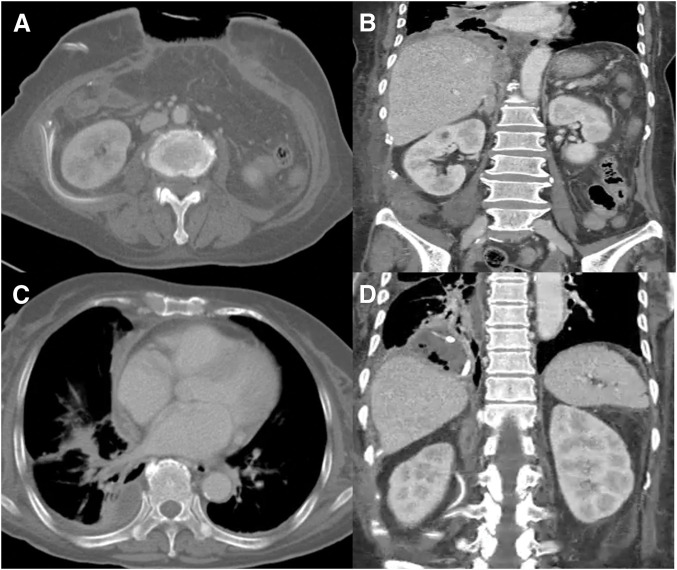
Follow-up CT images demonstrating interval improvement of the infectious process. Marked decrease in fluid collection at the operative site (A). Decrease in mediastinal and suprahepatic fluid collections (B). Reduction of pleural fluid with improvement of the empyema (C). Resolution of the pleural and mediastinal fluid collections with a patent PCD (D). PCD, percutaneous catheter drainage
